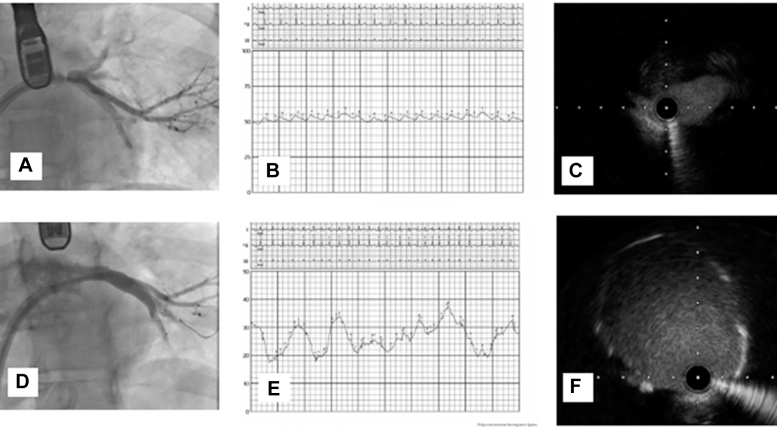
Prestenting and Poststenting Prestenting: (A) angiography with a severe LIPV lesion, (B) L PCWP = 53 mm Hg, and (C) IVUS at the site of the lesion. Poststenting: (D) angiography with resolution of the LIPV lesion, (E) L PCWP = 26 mm Hg, and (F) IVUS with a well-expanded, well-apposed stent. IVUS = intravascular ultrasound; L PCWP = left pulmonary capillary wedge pressure.
